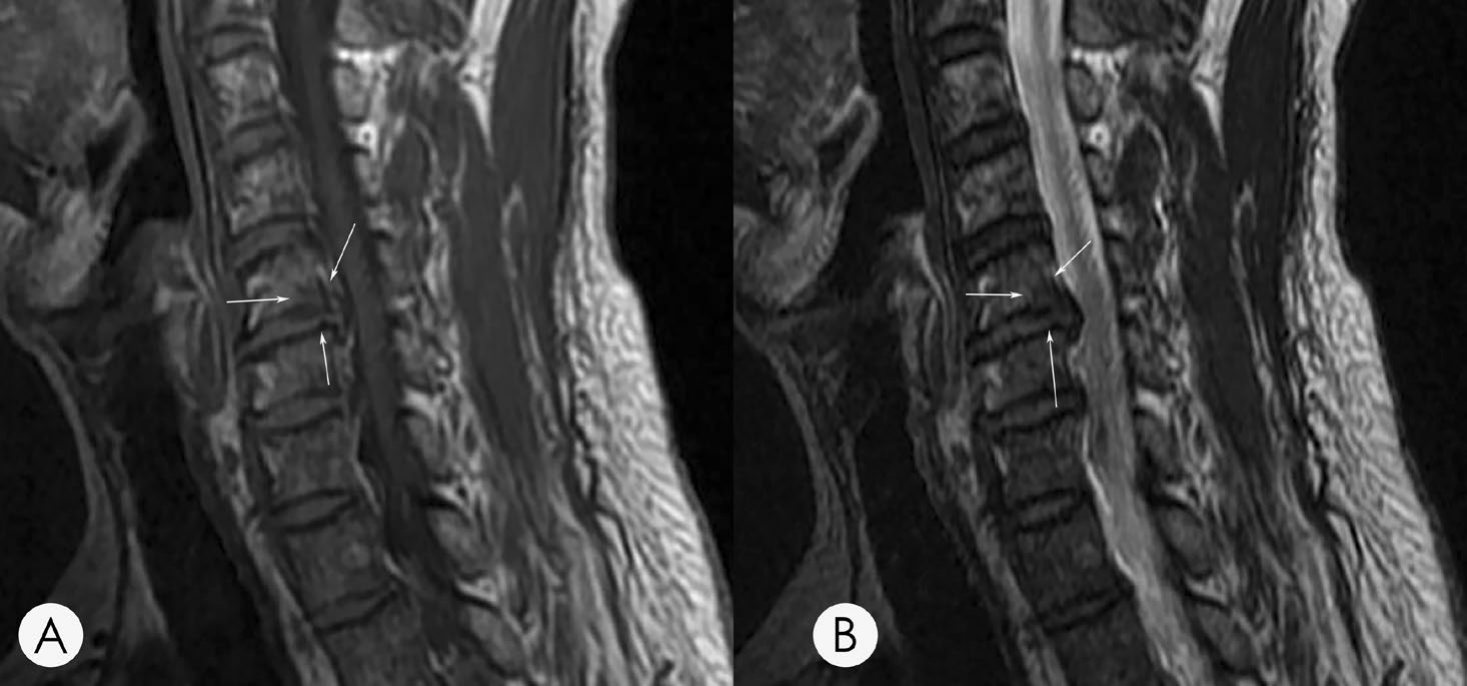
(A) A T1‐weighted magnetic resonance imaging (MRI) of a 51‐year‐old male demonstrates hypointensity at the inferior endplate of C5. (B) A T2‐weighted MRI of the same patient demonstrates hypointensity, suggesting a type 3 MC. Arrows indicate the location of the identified MC lesion on T1 and T2 imaging.
